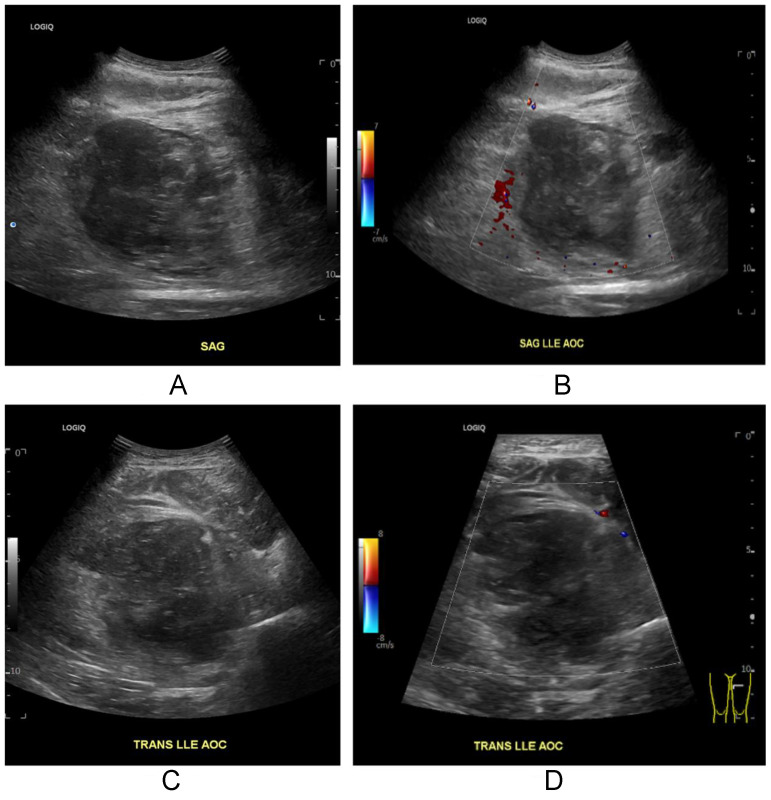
Sagittal grayscale (A) and color Doppler (B), and transverse grayscale (C) and color Doppler (D) ultrasound images of the left thigh demonstrate a heterogeneous intramuscular hematoma within the medial thigh musculature. No internal Doppler flow is identified within the hematoma.
